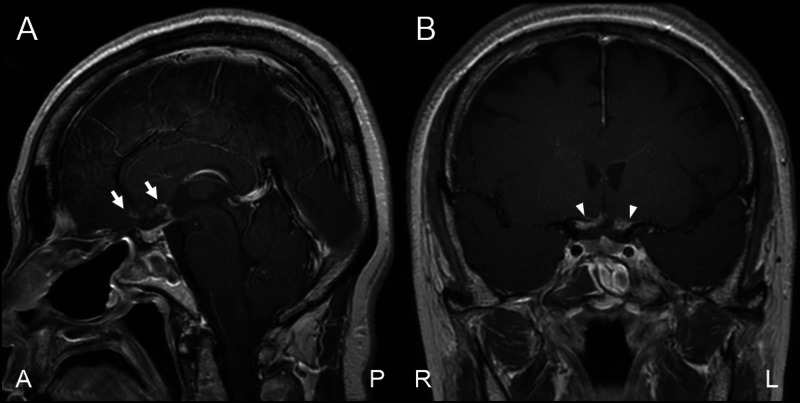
Postoperative MRI findings Sagittal and coronal contrast-enhanced T1-weighted images showed that almost all suprasellar tumors were resected (arrows) except at the lateral region and around the laminar terminalis region (arrowheads) (A, B) MRI: magnetic resonance imaging
